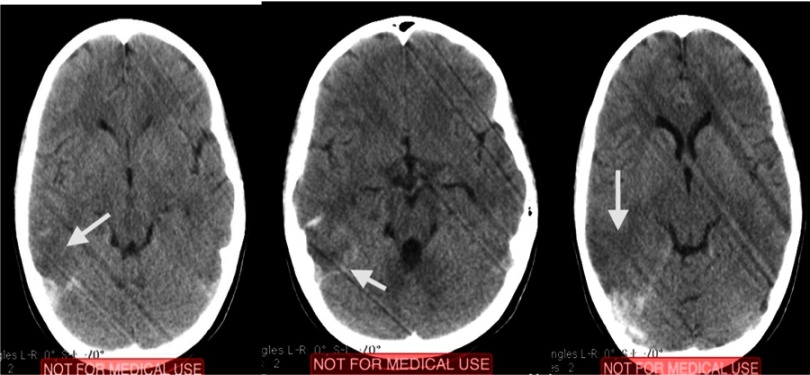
Initial CT scan, after the ictus. Arrows depict the areas of ischemic stroke (hypodense), with a surrounding area of hemorrhagic transformation.
